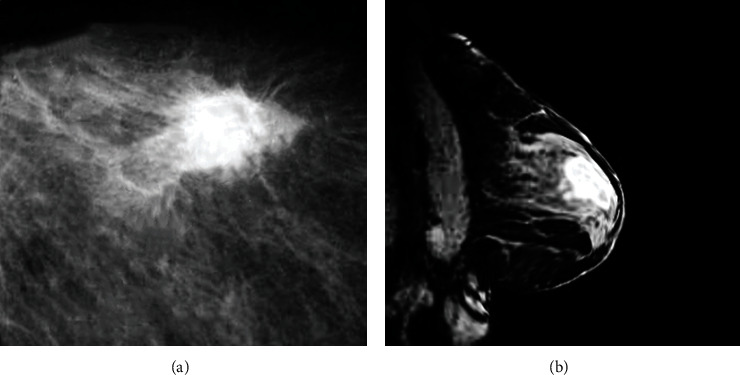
DCE-MRI images of two breast carcinoma patents: (a) lobular lumps; (b) annular enhanced lumps on the edge.
